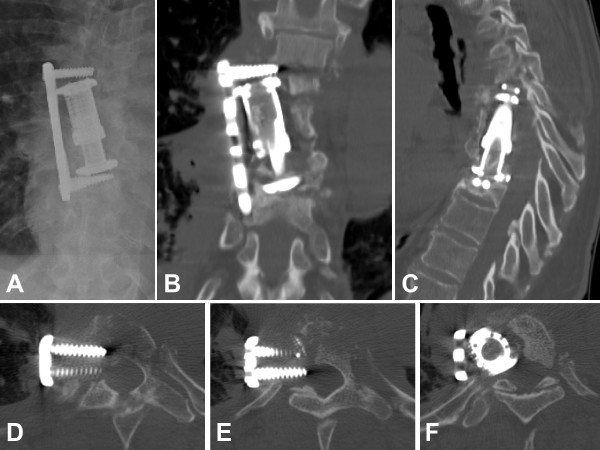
Failure of the initial anterior fixation at 6 months after surgery, secondary to repeat falls. Conventional anteroposterior X-ray (panel A) and coronal CT reconstruction (panel B) demonstrate the loss of fixation of the anterolateral locking plate and of the expandable cage, which are pulled out at the upper segment in T6. The sagittal CT reconstruction (panel C) demonstrates the kyphotic malunion cephalad to the expandabale cage. The axial CT views (panels D-F) shows the laterally pulled out locking head screws (D, E) and cage (F) at T6.
